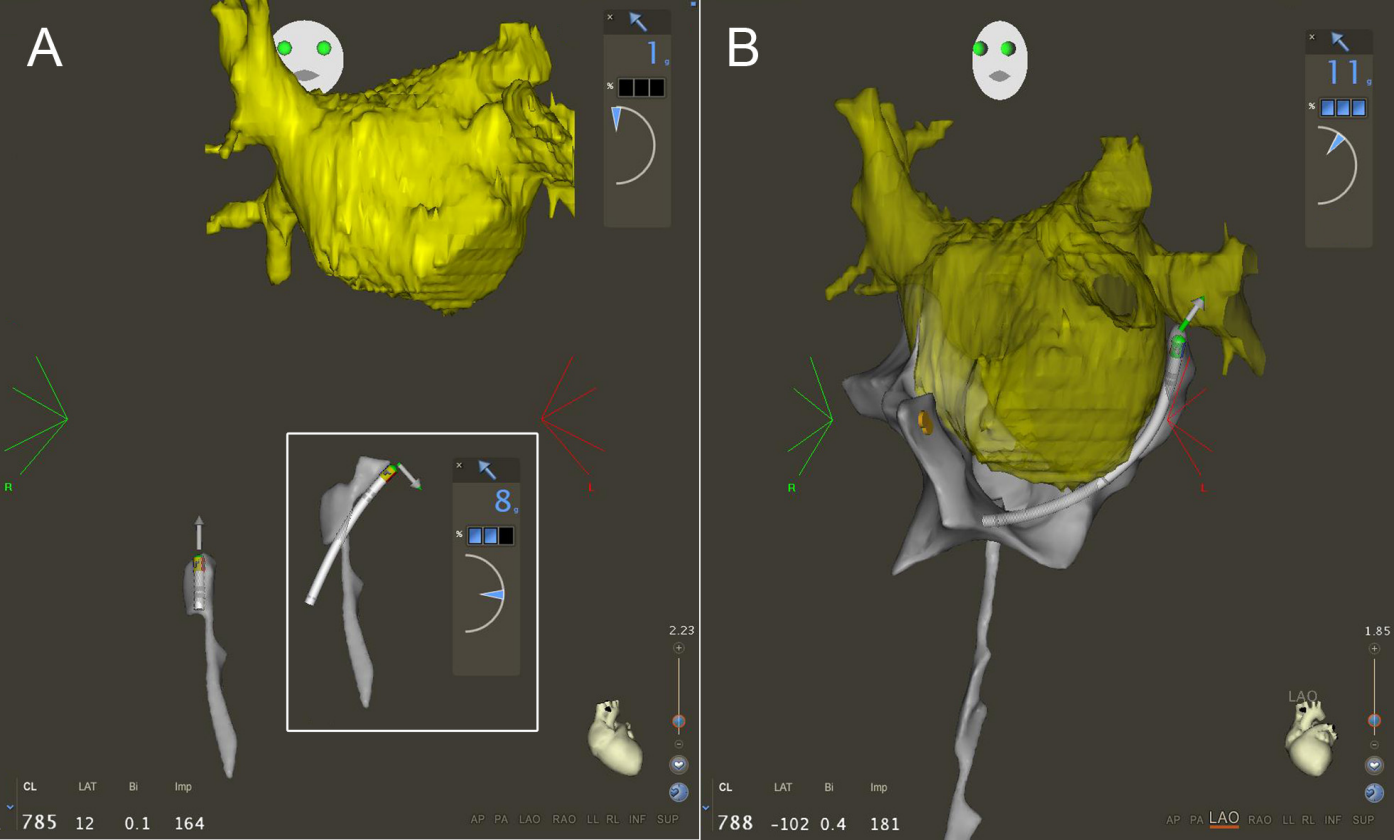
(A) Antero-posterior view of the fast anatomical map of the inferior vena cava. While advancing the catheter up to the right atrium, catheter tip orientation, force vector orientation, and contact force are monitored (outlined box). (B) Left anterior oblique view of the map of the right atrium with the 3D MRI reconstruction of the left atrium positioned based on the course of the coronary sinus and the septum. The yellow tag denotes the His position.
